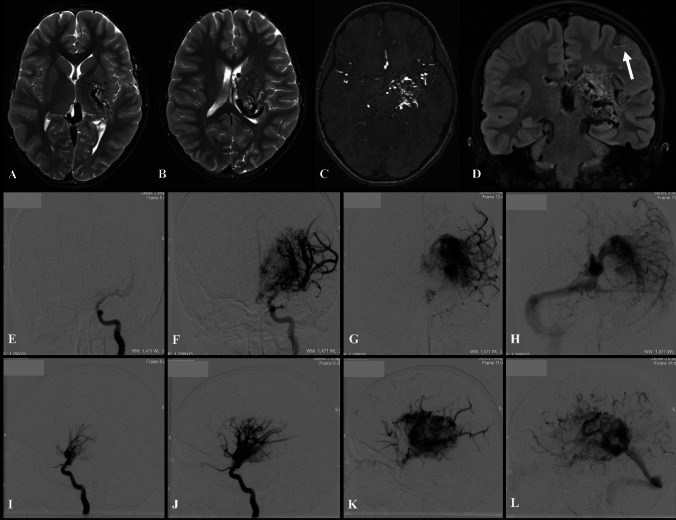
A, B T2-weighted MRI showing a left deep-seated thalamic/basal ganglia bAVM with deep venous drainage through left thalamo-striate vein towards the vein of Galen. Note the subtle prominence of cortical sulci and cerebrospinal fluid (CSF) spaces on the left frontal and parietal regions compared to the right hemisphere consistent with a subtle degree of volume loss. There is no direct encephalomalacic changes within the subcortical structures to suggest direct injury to the region. C MRA showing nidal component on the left thalamic/basal ganglia bAVM with supply from perforating vessels. D Coronal FLAIR MRI sequence showing Ivy sign in the left peri-Rolandic sulci. (arrows). E–H AP view of DSA after left ICA injection showing rapid shunting of the AVM nidus and early venous drainage. E, F There is an arterial supply predominantly from MCA branches (left lenticulo-striatal, Heubner) and anterior choroidal artery. I–L Lateral view of the DSA evidencing the AVM supply by the anterior choroidal (I) and lenticulo-striatal vessels (J). Notice also the paucity and slow filling of the cortical hemispheric vasculature as a result of the high-flow and rapid bAVM shunting
